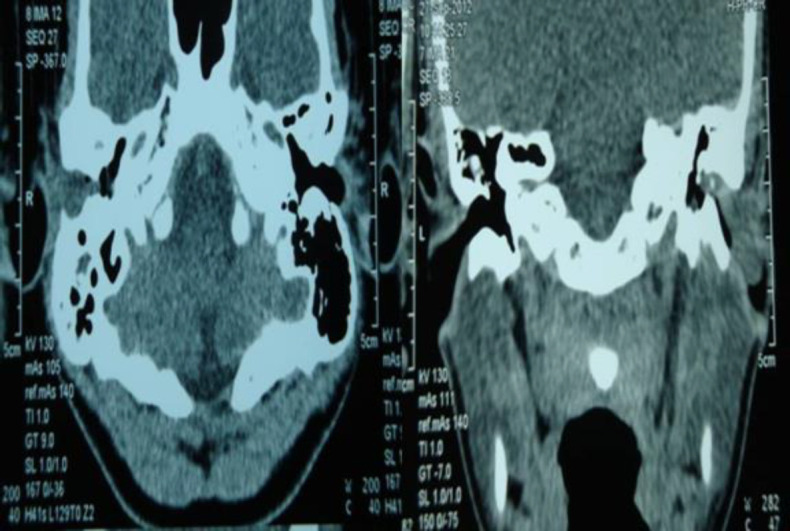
CT scan in axial and coronal view, showing soft tissue mass in the external auditory canal without bony erosion or involvement of the tympanic membrane
